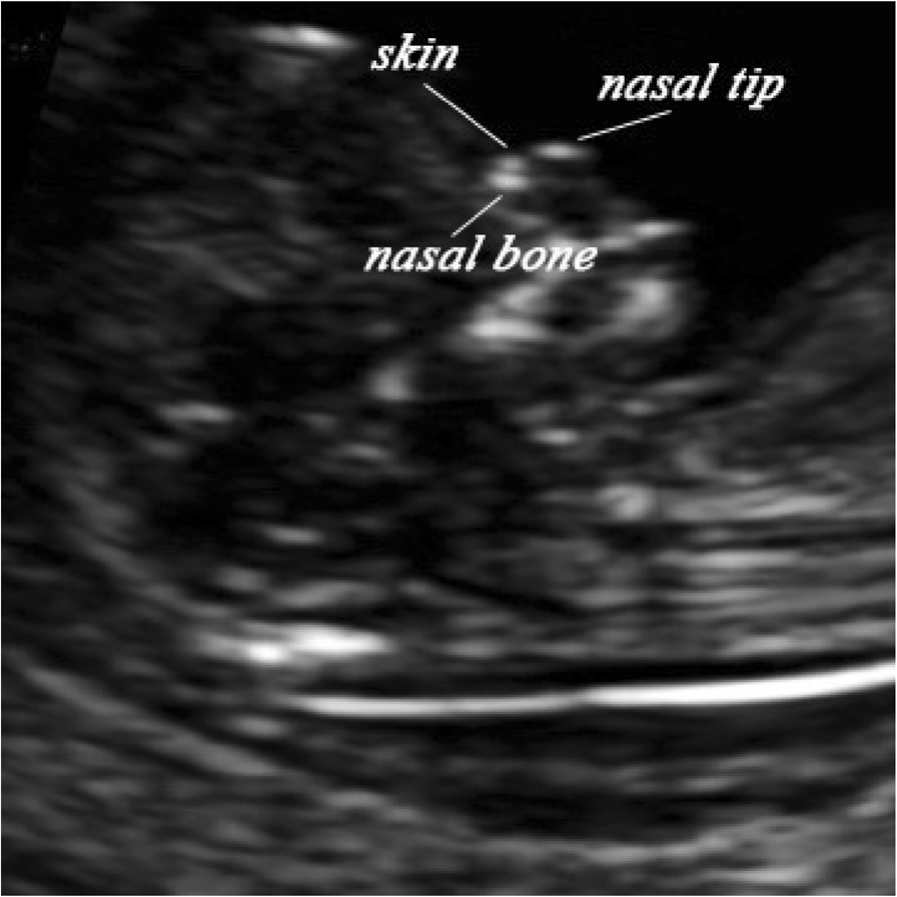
Ultrasound image in the midsagittal view showing nasal bone, nasal skin and tip of nose.
